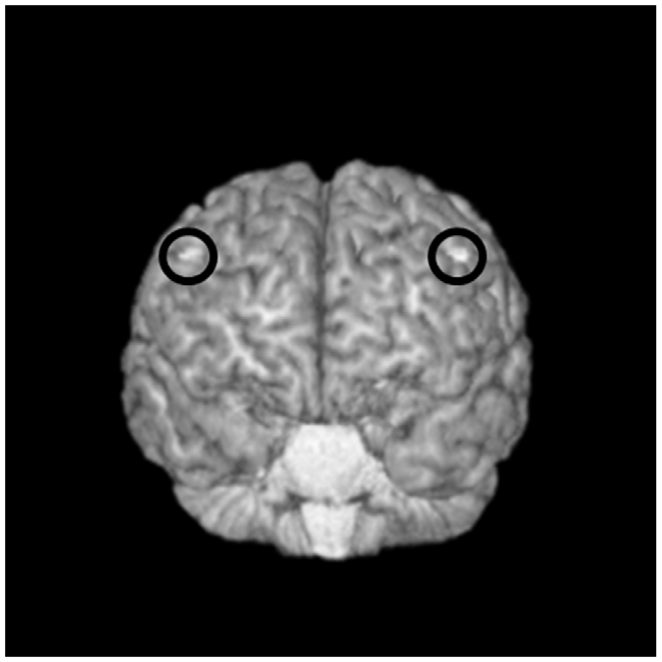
Targeting the Dorsolateral Prefrontal Cortex (DLPFC) for rTMS stimulation. Transverse view from a single subject with exposed cortex and overlap of Brodmann areas 9 & 46, highlighted (white) on a T1-weighted 3D MRI. Using MRI-to-MiniBird co-registration, the centre of the TMS coil was held over this region.
